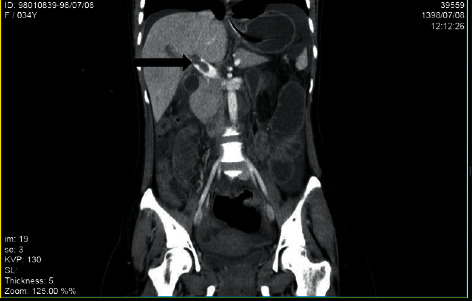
Thrombosis in main portal vein with its extension to right and left intrahepatic branches in spiral abdominal CT scan with IV contrast (black arrow).
